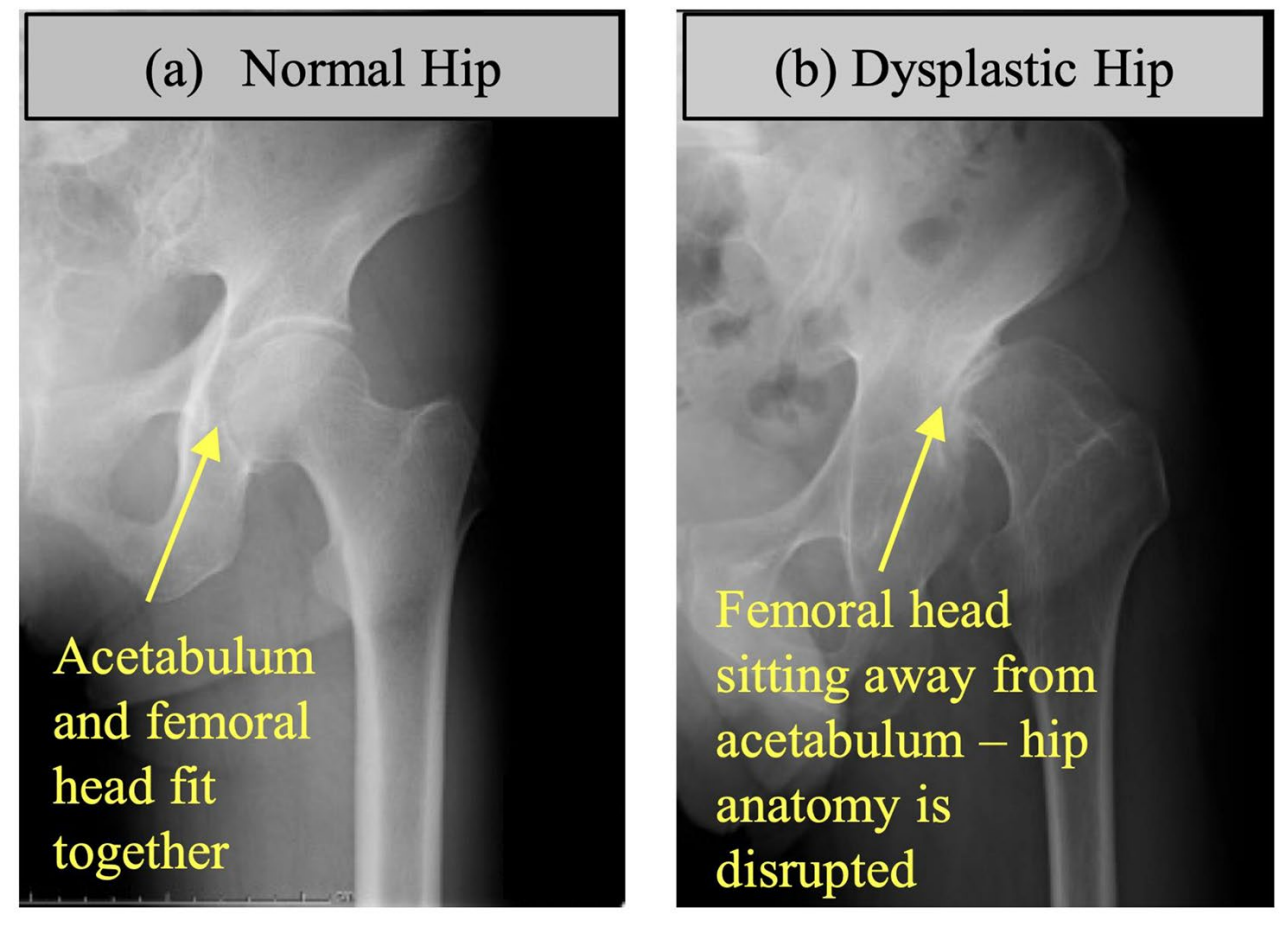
Examples of (a) normal, and (b) dysplastic hip radiograph
